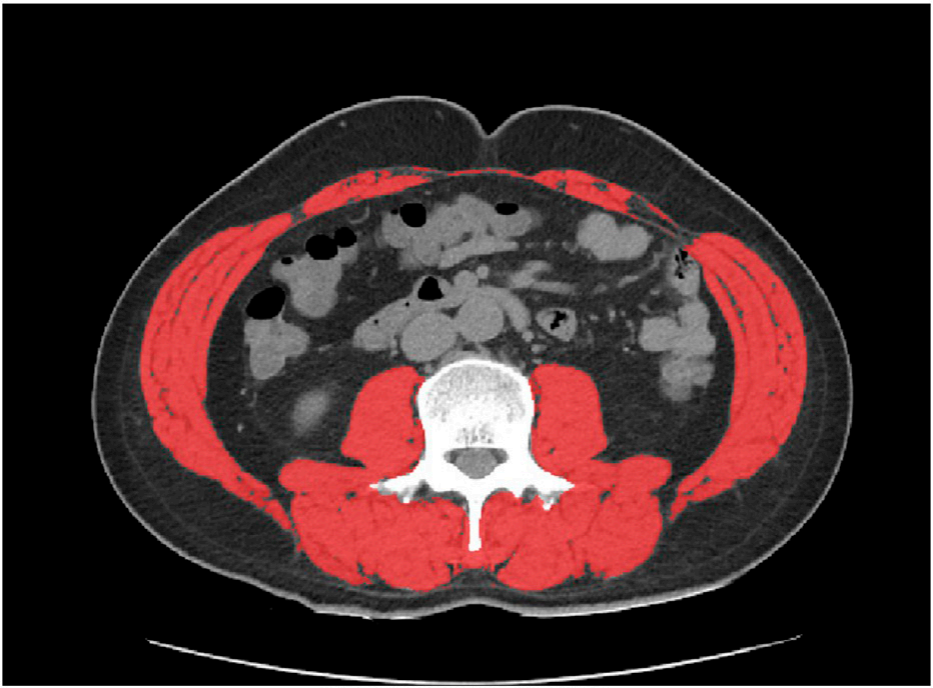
CT images of L3 skeletal muscle area measurement (red area represents skeletal muscle area).
